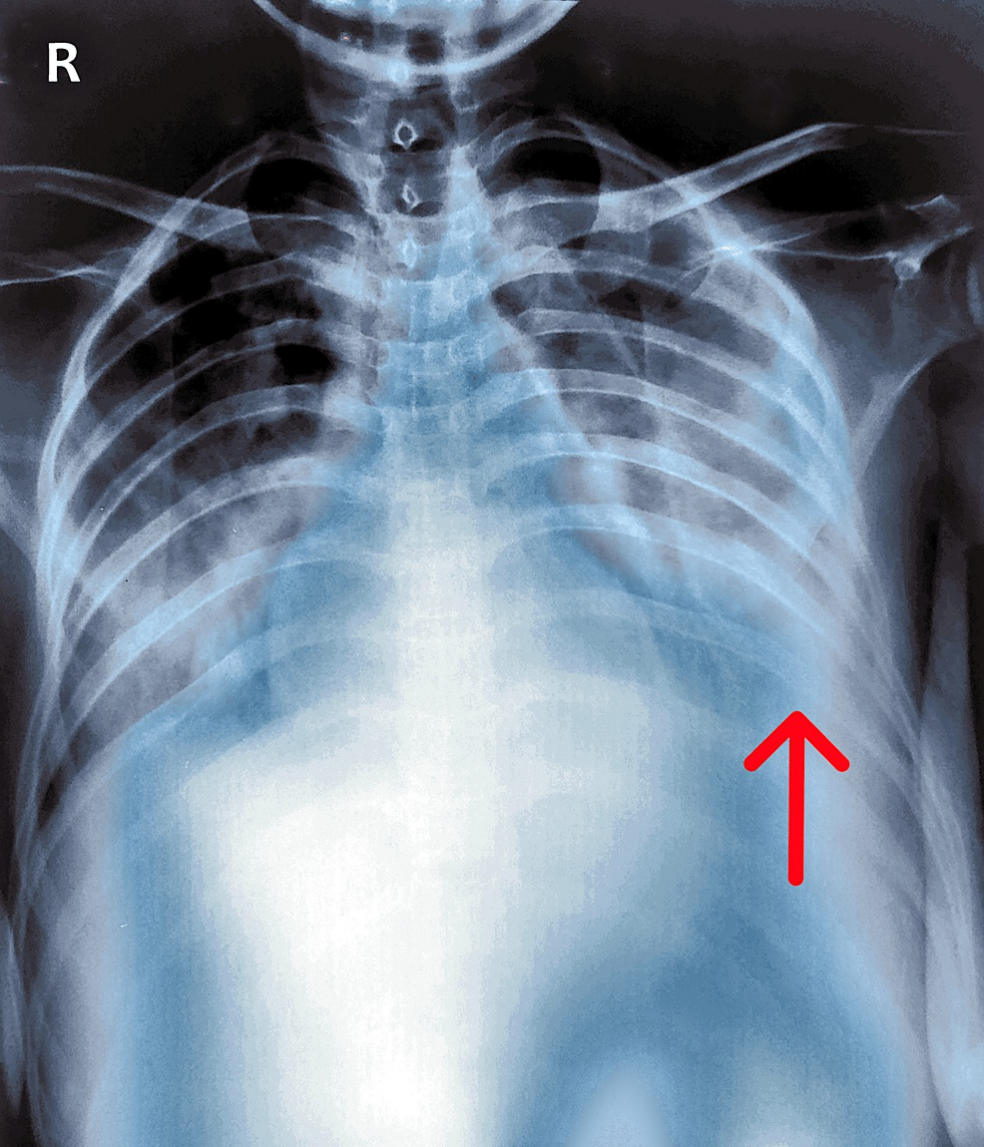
A chest x-ray anterior-posterior view showing heterogeneous opacities with air bronchograms in bilateral lung fields and bilateral pleural effusion, left more than right
